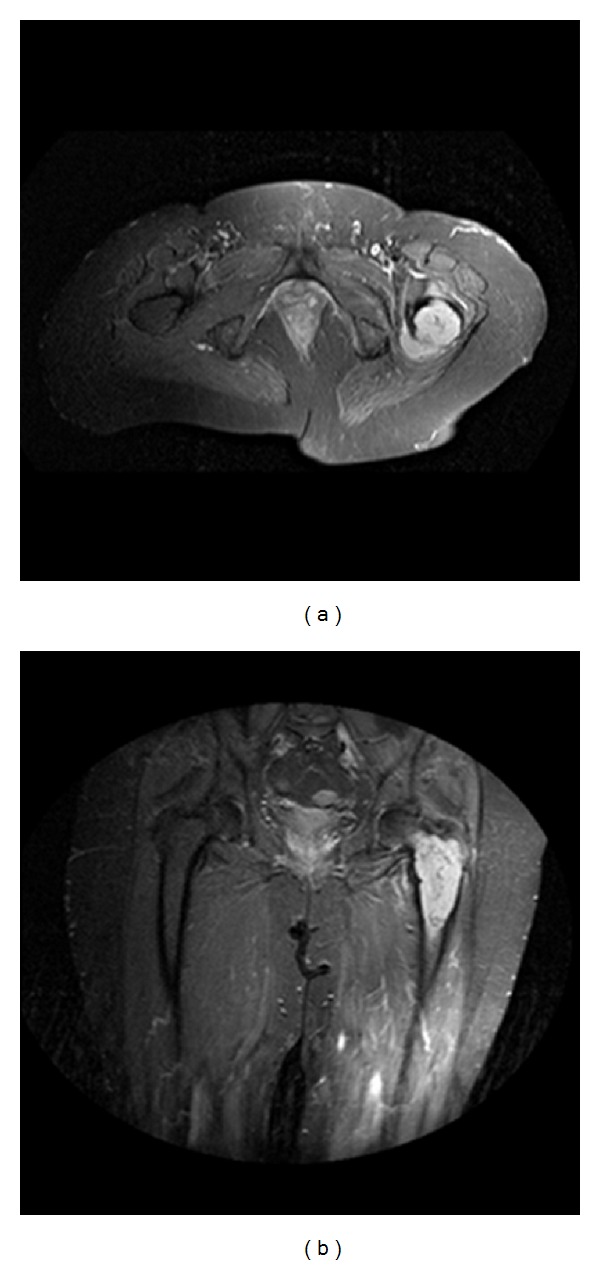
Preoperative MRI showing the left femur of a 76-year-old Caucasian female with diagnosis dedifferentiated chondrosarcoma (a) anterior-posterior axial view, (b) anterior-posterior front view.
